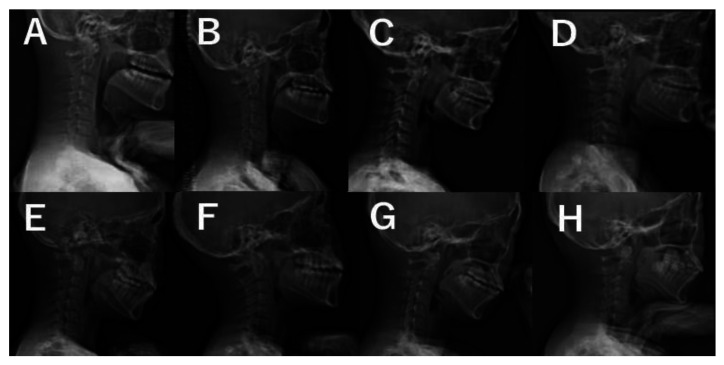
Pre- (A,C,E,G) and post- (B,D,F,H) operative magnified radiographs of the cervical spine from the cases in Figure 1 (A,B), Figure 2 (C,D), Figure 3 (E,F), and Figure 4 (G,H).
